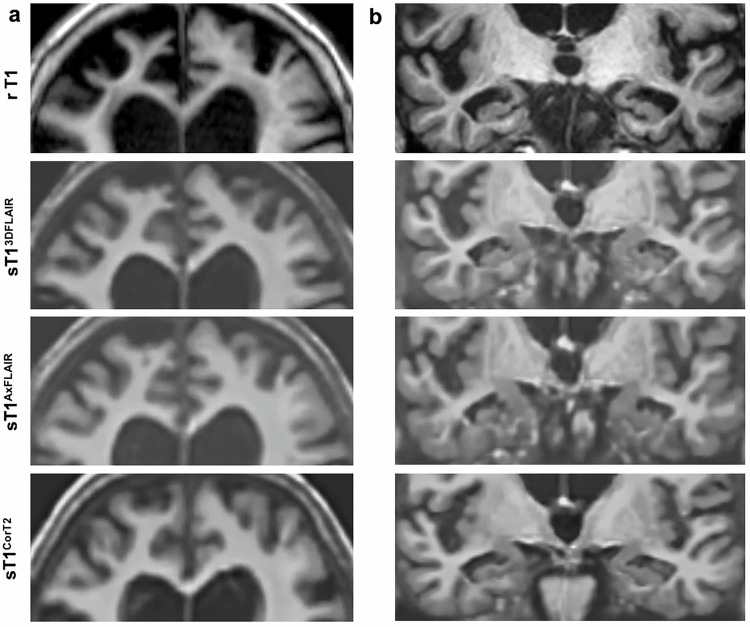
Real 3D T1 and SynthSR generated 3D T1w images from the different input sequences of the frontal lobe of one patient with FTD (a) and the Hippocampus from one patient with Alzheimer’s Disease (b). In (a) partial volume effect of the parenchyma is evident in all SynthSR-generated images, particularly pronounced in  sT1^corT2^. rT1, real 3D T1; sT1^3DFLAIR^, SynthSR generated 3D T1 from 3D FLAIR input; sT1^AxFLAIR^, SynthSR generated 3D T1 from axial FLAIR input; sT1^CorT2^, SynthSR generated 3D T1 from coronal T2 input
